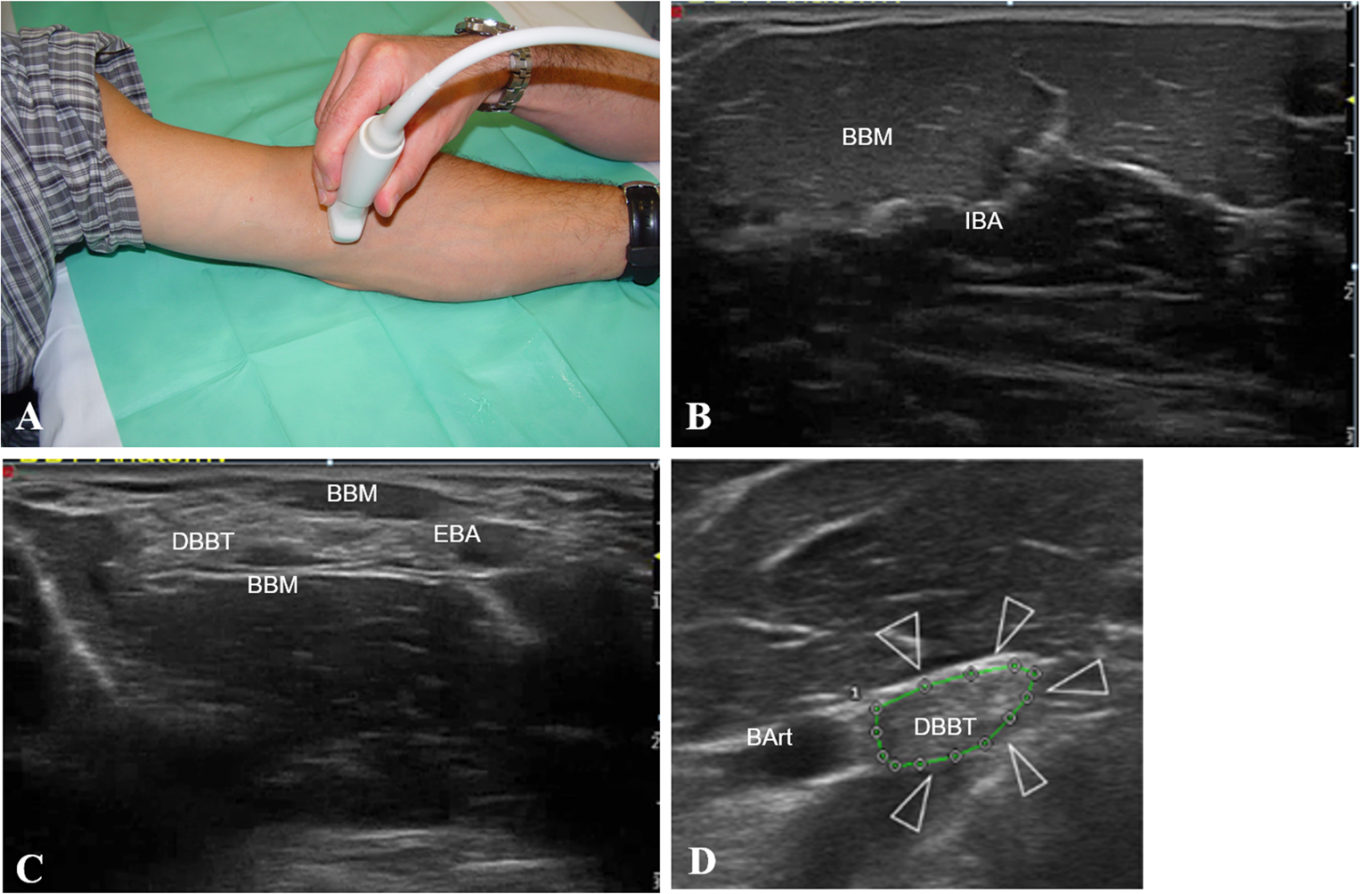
Short-axis anterior approach. a The patient is sitting on a stool in front of the examination table resting the elbow in full extension and forearm in full supination. The ultrasound transducer is placed in the axial plane on the anterior half of the arm and then shifted distally. b Intramuscular region: ultrasound cross-section view of the internal bicipital aponeurosis (*IBA*) centred within the biceps brachii muscle (*BBM*) belly. c Transition region: the distal biceps brachii tendon (*DBBT*) has transitioned to an oval shape, the external bicipital aponeurosis (*EBA*) is observed extending medially with some muscle fibres remaining. It corresponds to the terminal part of the myotendinous junction. d Free-tendon: the oval-shaped DBBT has transitioned to a deeper plane towards the radial tuberosity (not visible on the image), and is found lateral to the brachial artery (*BArt*)
